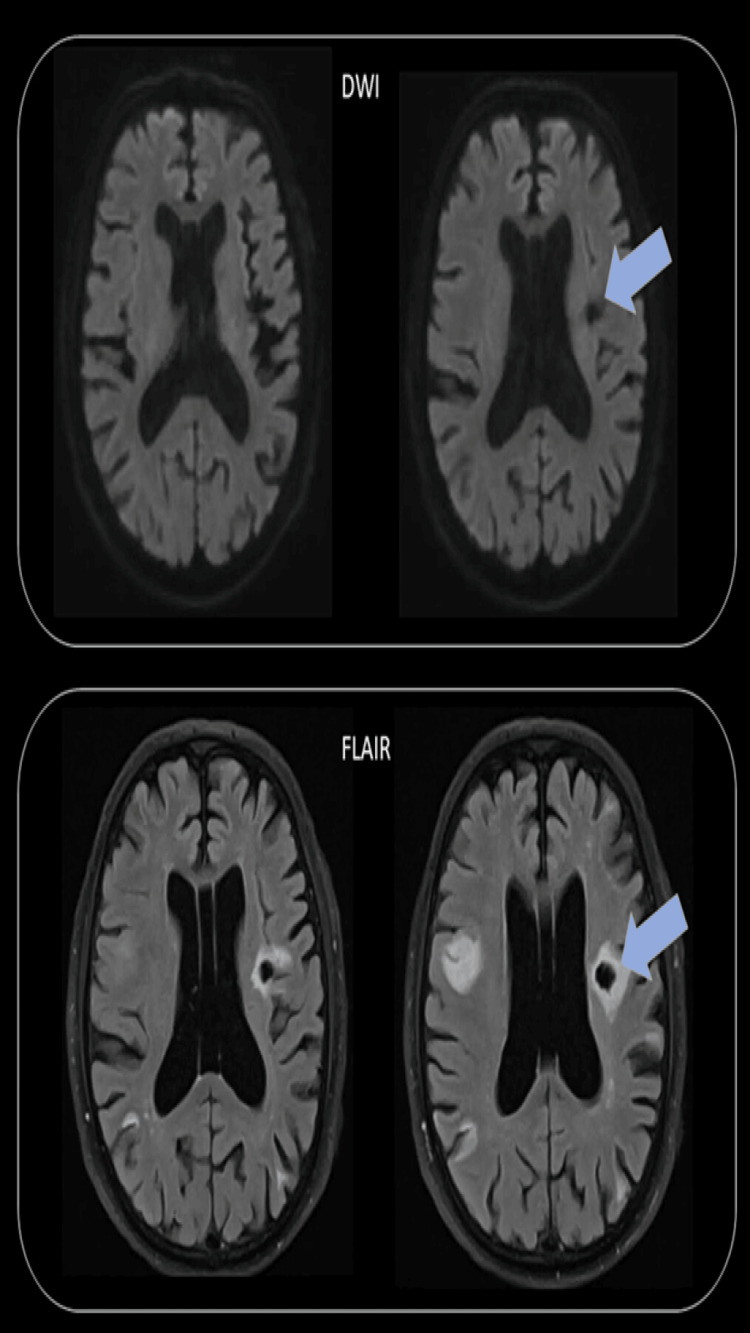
MRI brain diffusion-weighted imaging (DWI) and fluid-attenuated inversion recovery (FLAIR). Post-stenting. (Arrow) demonstration of chronic lacunar infarctions.
